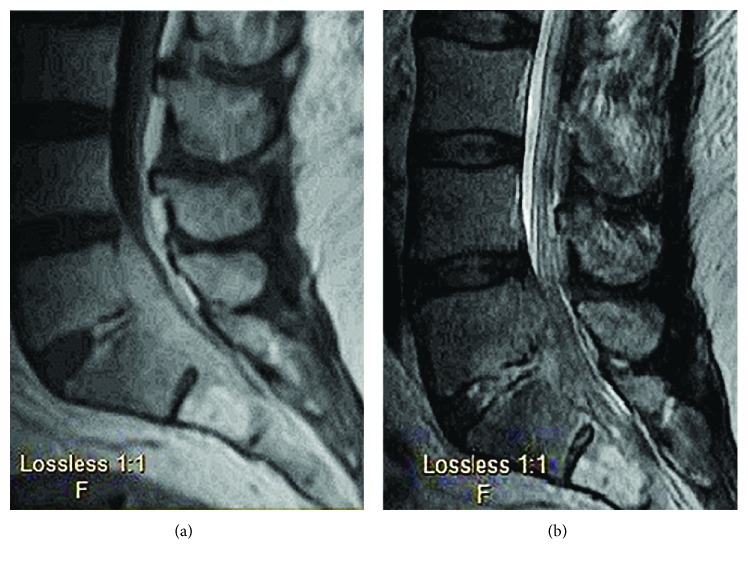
Post-T1- and T2-weighted MRI of lumbar spine upon hospital admission. Confirmed suspected discitis, osteomyelitis with end plate destruction, and epidural abscess seen on CT.
